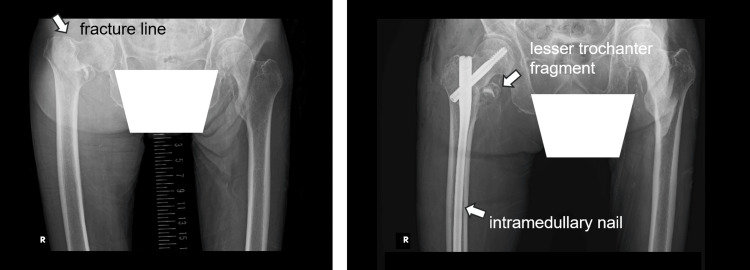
Radiographic findings. (A) Preoperative radiograph showing the right hip fracture.
